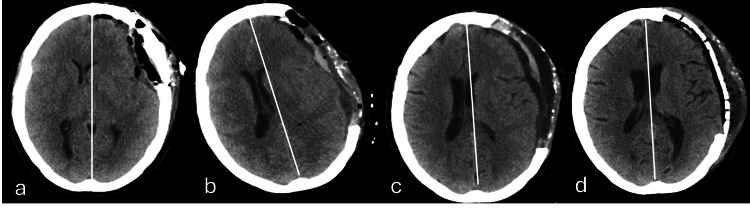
Chronological axial plane of brain computed tomography obtained throughout the clinical course (a) Post-meningioma resection. (b) Midline deviation of the structures to the right after decompressive craniectomy (herniation to the opposite site of the cranial defect). (c) Reduction of the herniation mass effect after adopting measures to increase the intracranial pressure. (d) Cranioplasty with plate (PEEK). The white line represents the midline. PEEK, polyetheretherketone
